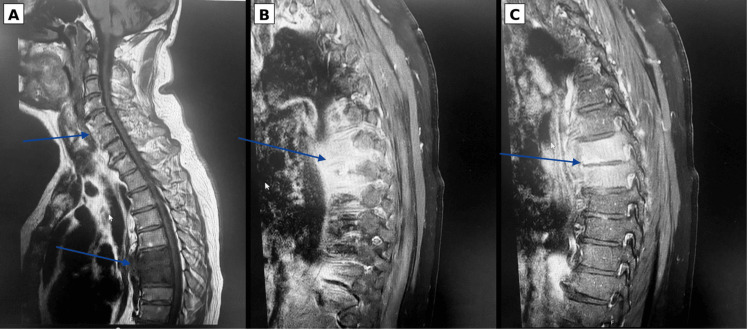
MRI Whole-spine MRI with contrast, 24 December 2025. Sagittal views. (A) Overview through the cervicothoracic spine, with loss of disc height and altered marrow signal at T6–T7. (B) Fluid-sensitive sequence through the thoracic spine, showing marked marrow oedema in T6 and T7, destruction of the intervening disc, and prevertebral inflammatory signal. (C) Post-contrast sequence with fat saturation, with avid enhancement of both vertebral bodies and the intervening disc, plus enhancement of the adjacent prevertebral soft tissues extending anteriorly. Appearances were those of severe T6–T7 discitis and osteomyelitis. The affected vertebral level was reported as T7–T8 on CT angiography and T6–T7 on MRI; this difference reflects the lower spatial resolution of CT for early endplate change rather than progression of disease
